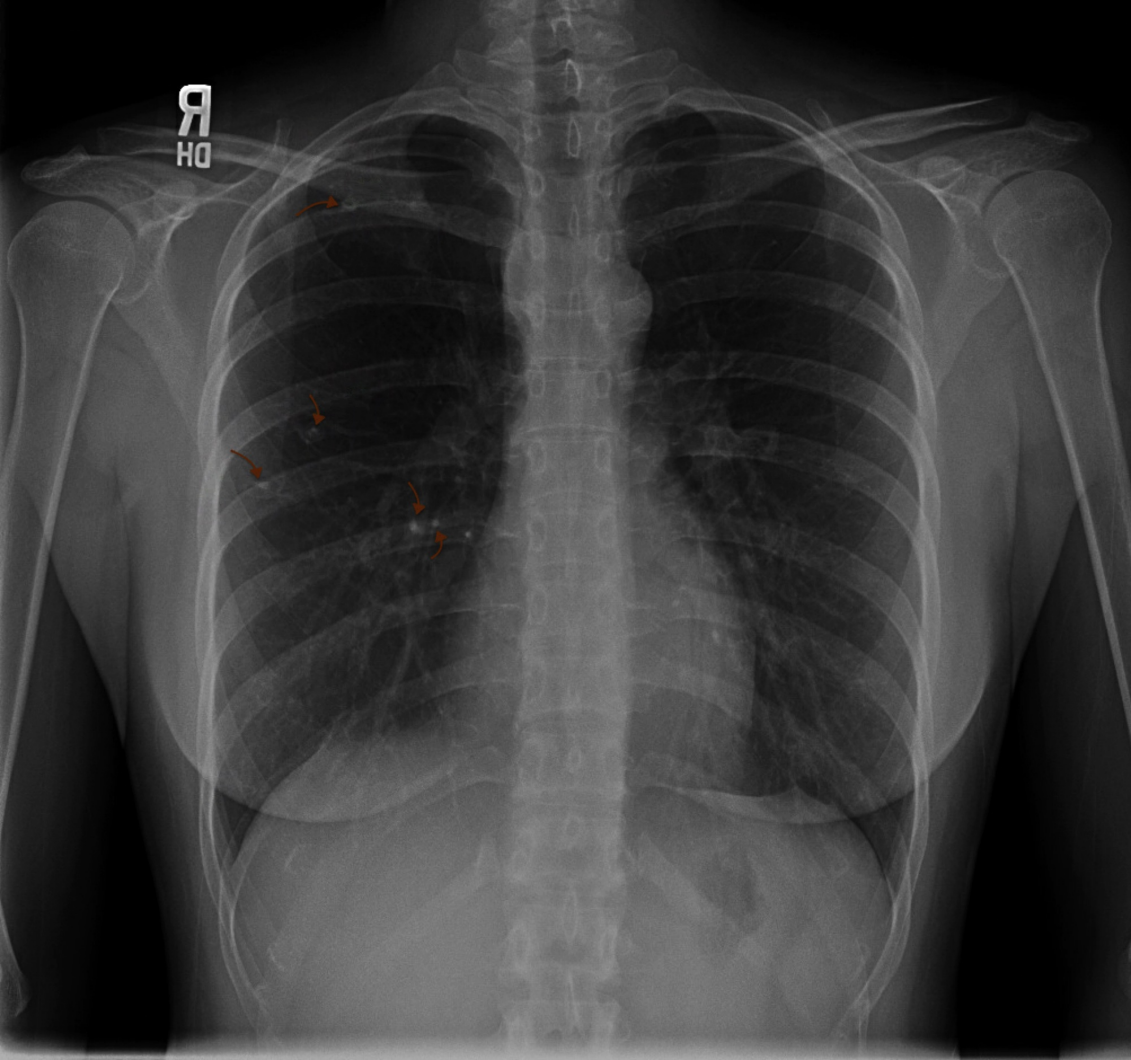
Chest X-Ray There were small rim calcified lesions in the right mid and upper lung zones suggestive of a benign etiology without findings of active or latent tuberculosis infection. R: right side.
